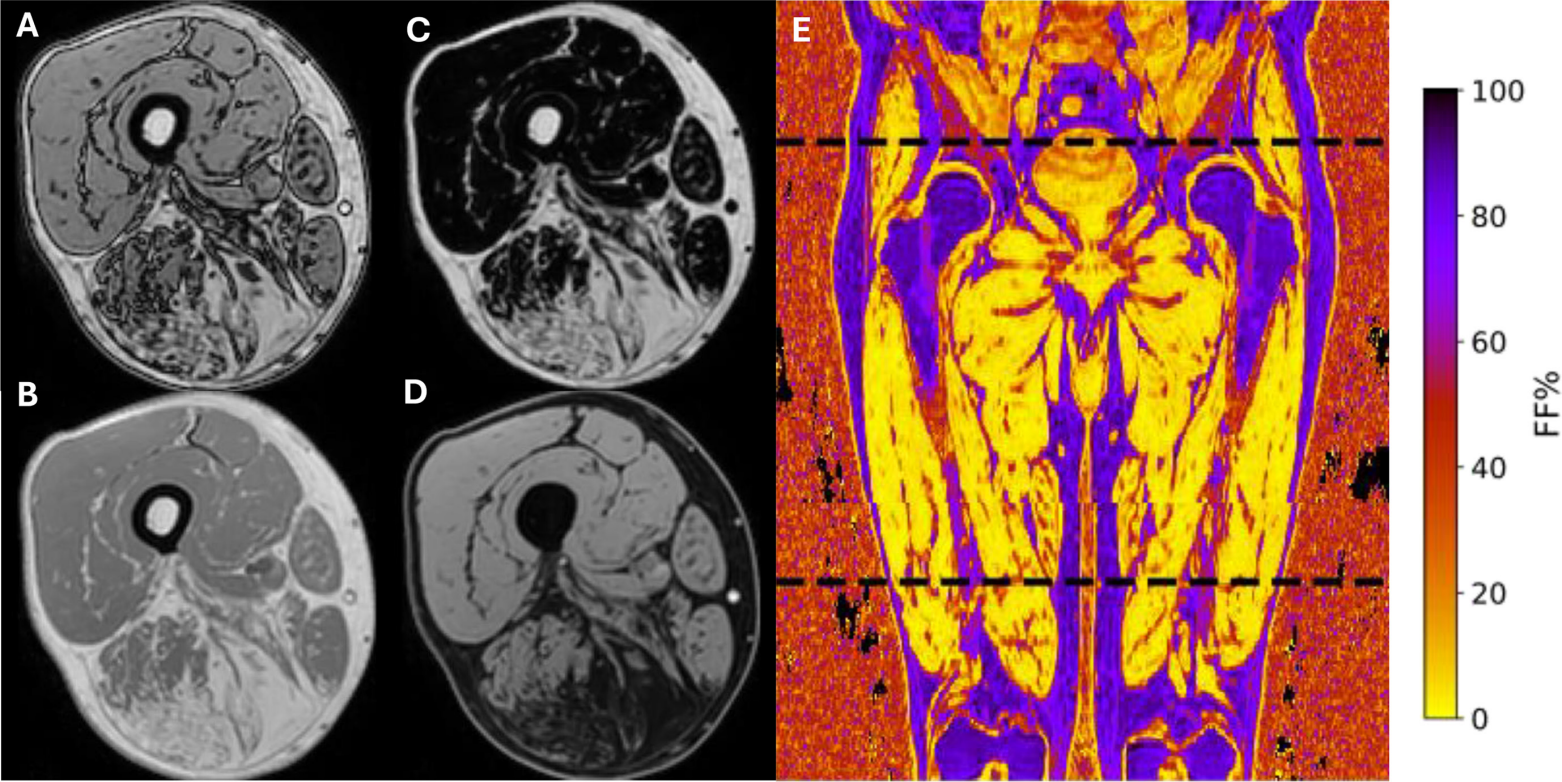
Dixon MRI images. Axial slice of the out‐of‐phase (A), in‐phase (B), fat‐only (C) and water‐only (D) images of a LGMDR12 patient and coronal slice (E) showing the PDFF image with the full field of view. The limited field of view is indicated by the black dotted lines.
